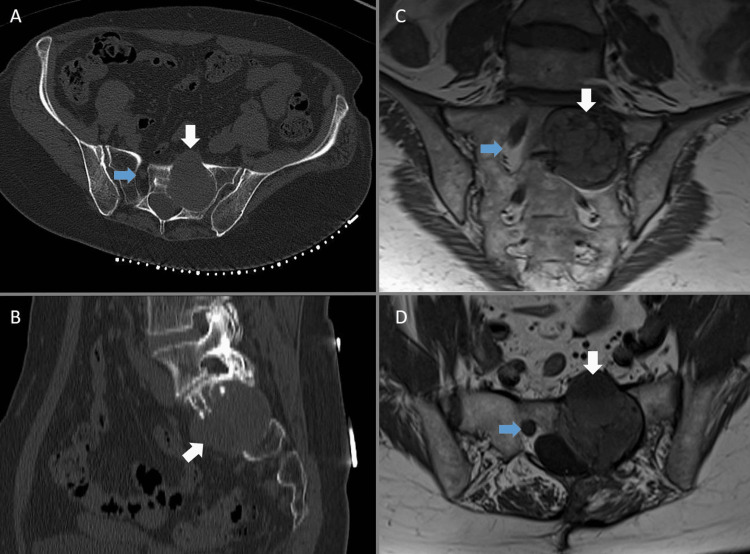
Case three Sixty-one-year-old female with malignant peripheral nerve sheath tumor originating from the left S1 nerve root. Axial and sagittal CT views (A and B) demonstrate a lytic left sacral mass centered on the left S1 foramen. Further imaging with coronal and axial T1 MRI is included in images C and D. The tumor is marked by the white arrows. The normal right S1 foramen for comparison is marked by the blue arrows.
